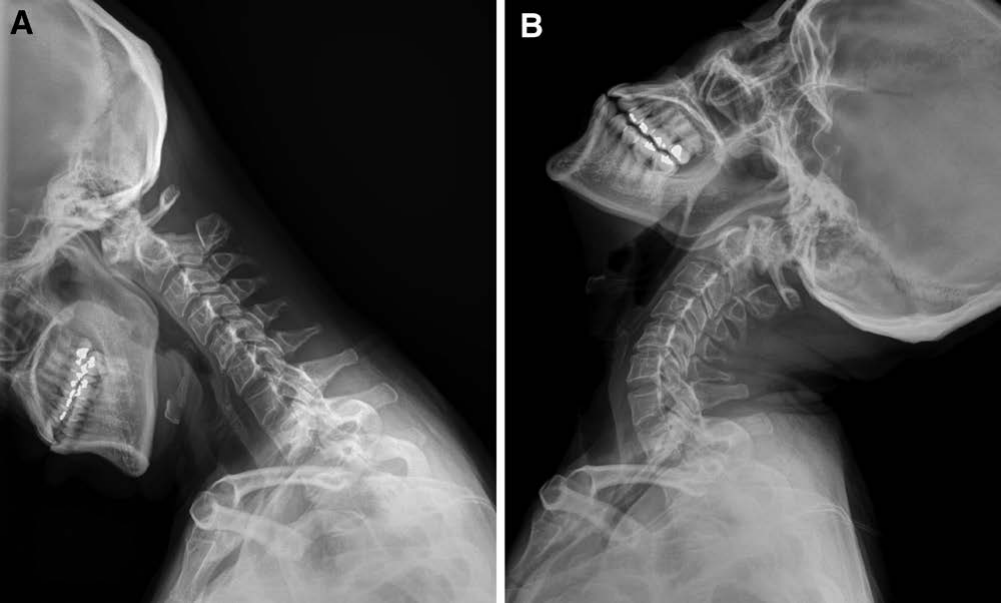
(Case 2) Plain dynamic flexion–extension radiographs show flexion (A) and extension (B) positions with subluxation on cervical flexion.
